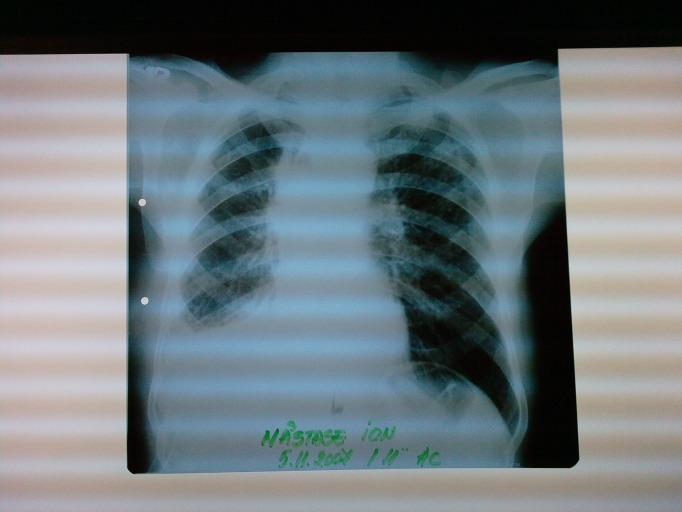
Postoperative chest X-ray – complete reexpansion of the right lung
